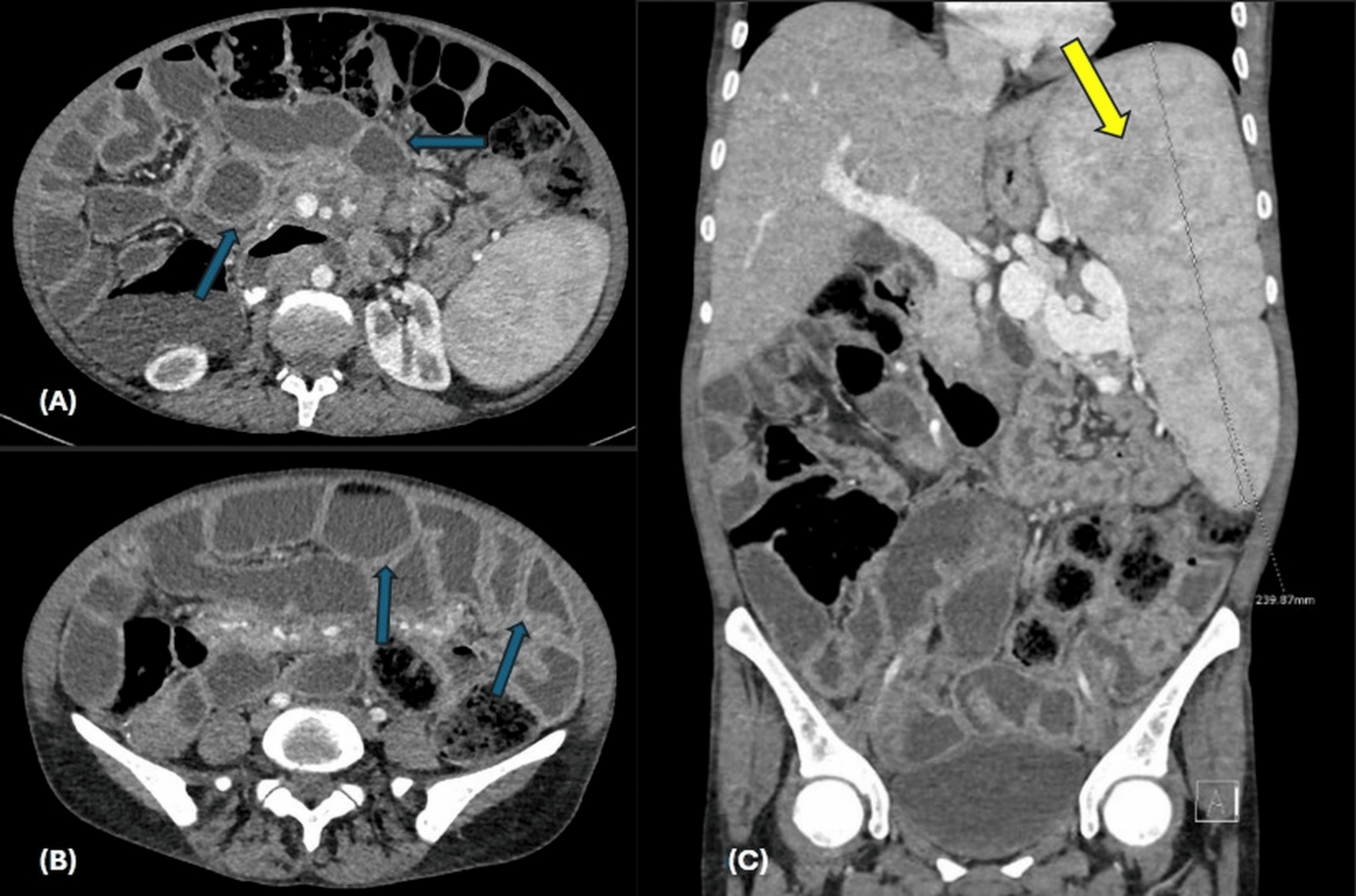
Contrast-enhanced CT enterography Axial images (A) and (B) and a coronal image showing diffuse circumferential mural small bowl wall thickening (blue arrows), as well as an enlarged spleen measuring 24 cm (yellow arrow).
